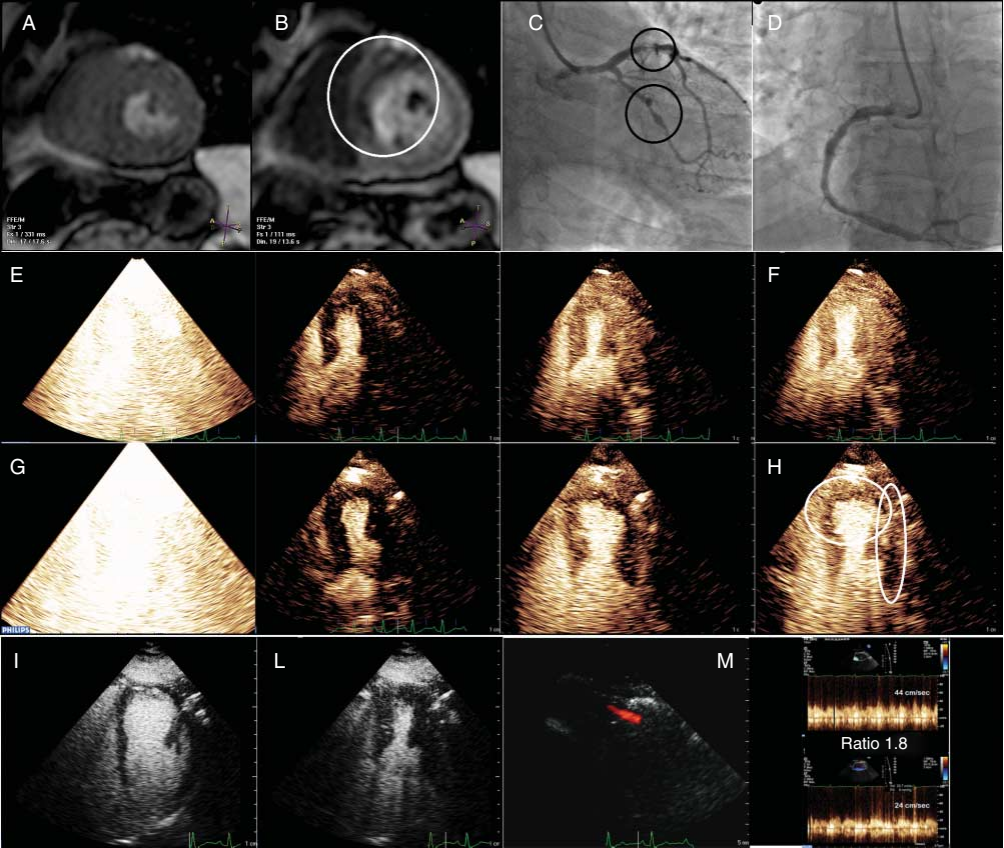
(A) aCMR rest short axis view. (B) aCMR stress short axis view. (C) Coronary angiography (LAD and circumflex arteries). (D) Coronary angiography (RCA). (E) Echocardiography: four-chamber view (flash-replenishment sequence at rest depicted from left to right). (F) Echocardiography: four-chamber view (image acquired 4 s after flash, indicating normal myocardial replenishment). (G) Perfusion defects in the lateral and apical segments after dipyridamole. (H) Still frame acquired 2 s after flash, white ovals indicate transmural lateral and subendocardial apical defects. (I) Wall motion at stress, end-diastolic image. (L) Wall motion at stress, end-systolic image. (M) Stress/rest Doppler diastolic velocity ratio on the LAD (color Doppler and pulsed-wave tracings).
